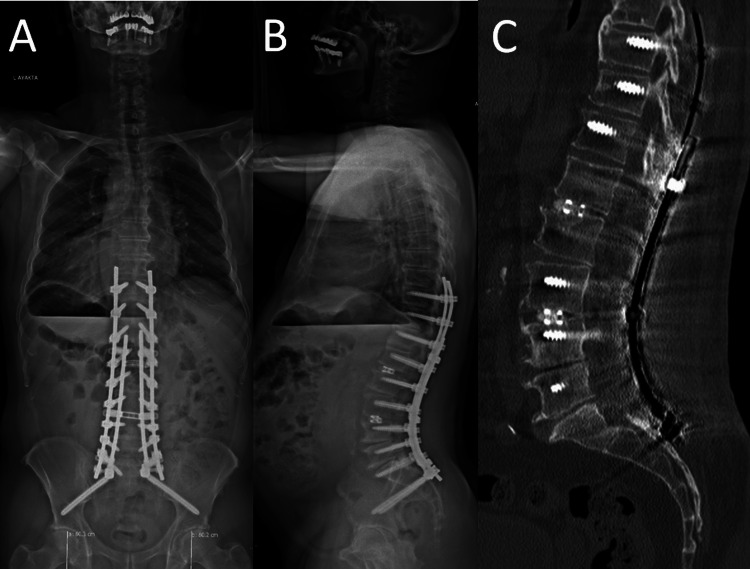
(A) Postoperative X-ray demonstrating T10-S2 posterior instrumentation. (B) Postoperative lateral X-ray demonstrating T10-S2 posterior instrumentation, preferred sagittal contoured rods, and interbody fusion of L2-L3 and L4-L5 levels. (C) Postoperative sagittal computed tomography (CT) scan demonstrating L1-L5 total laminectomy and interbody fusion of L2-L3 and L4-L5 levels.
